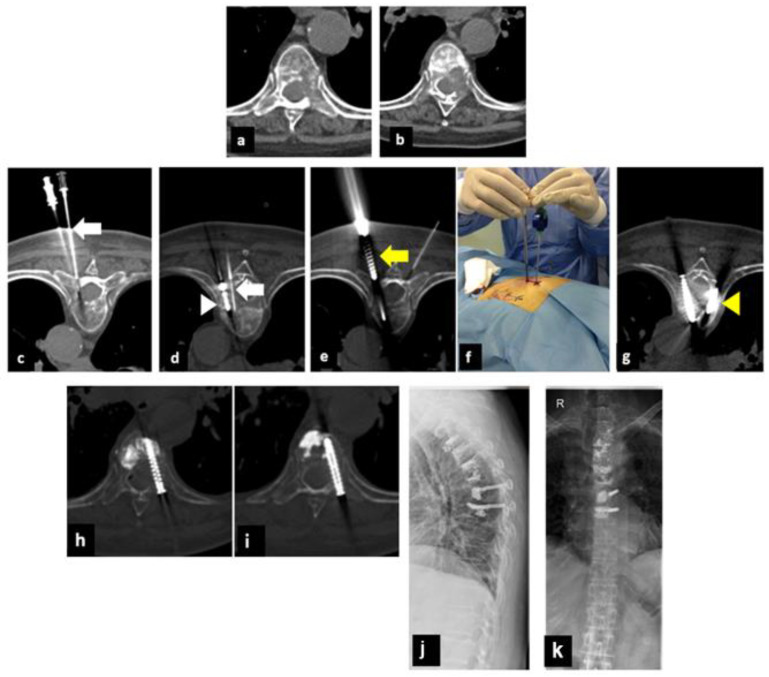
A 67-year-old woman with a history of non-small cell lung cancer, previously treated with multiple dorsal vertebroplasties for pathological vertebral fractures. (a,b): Axial CT shows extensive osteolytic metastases of the D7 and D8 vertebral bodies that involved the right pedicle in both cases. (c,d): Axial CT shows thermocouple placement (white arrows) and thermal ablation with MW (arrowhead). (e,f): Axial CT scan and intraoperative image (f) show the positioning of the screw (yellow arrow). (g): Axial CT scan shows contralateral placement of the vertebroplasty cannula. h-i-l-m: Axial CT scan shows post-procedure control (h,i) and a radiograph of the thoracolumbar spine at 3 months in antero-posterior and latero-lateral projection (j,k) after microwave ablation combined with pedicle screw fixation followed by vertebroplasty (MASFVA).
